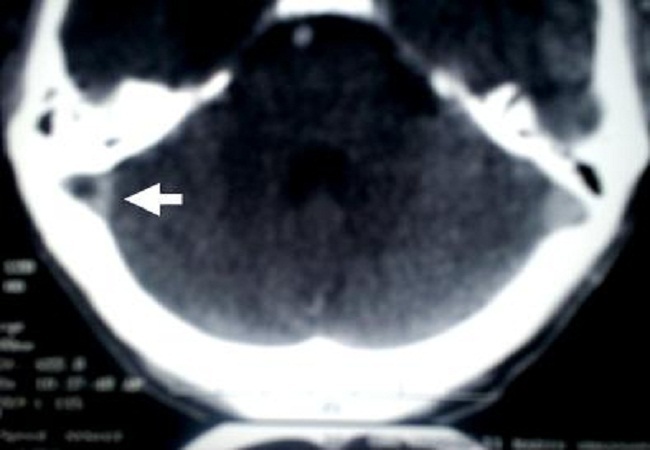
(case 14) CT scan after contrast product injection, axial sequence of the posterior fossa showing empty right lateral sinus (arrow)
